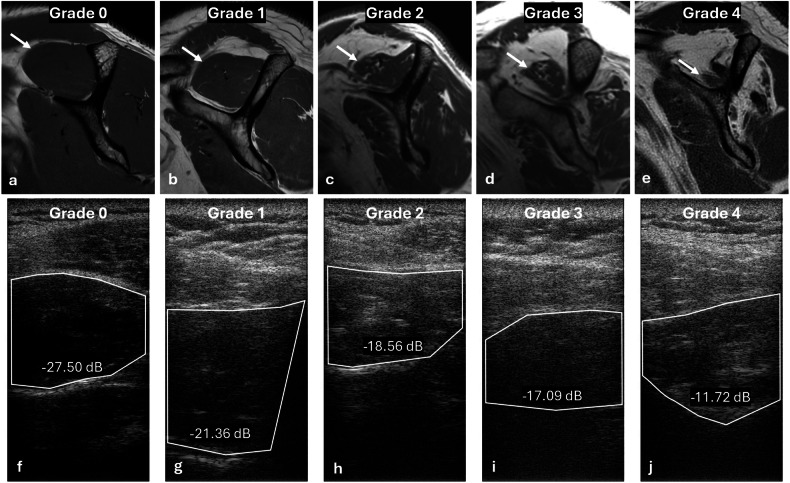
Representative images showing the range of MRI Goutallier grades (a–e) in the supraspinatus muscles (arrows) with the corresponding ultrasound images (f–j) and calculated backscatter coefficients from the regions of interest. Notice that the backscatter coefficient values increase with higher grades, however, the echogenicity on the B-mode images does not follow the same trend. B-mode echogenicity is determined by a variety of variables, including depth, whereas overlying tissue attenuation is corrected with quantitative ultrasound. MRI, Magnetic resonance imaging
